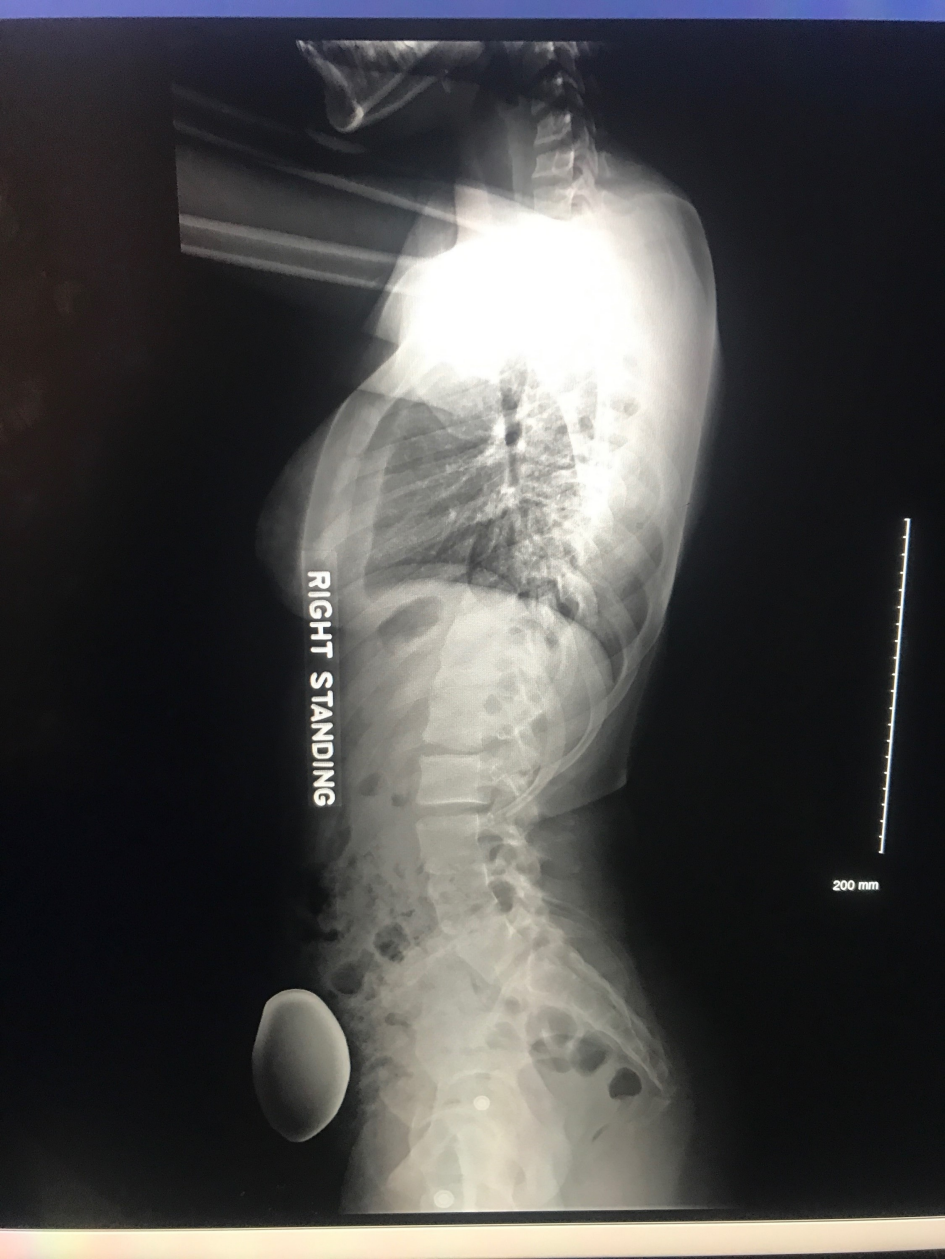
Preoperative lateral view of 36-inch standing scoliosis radiograph.
